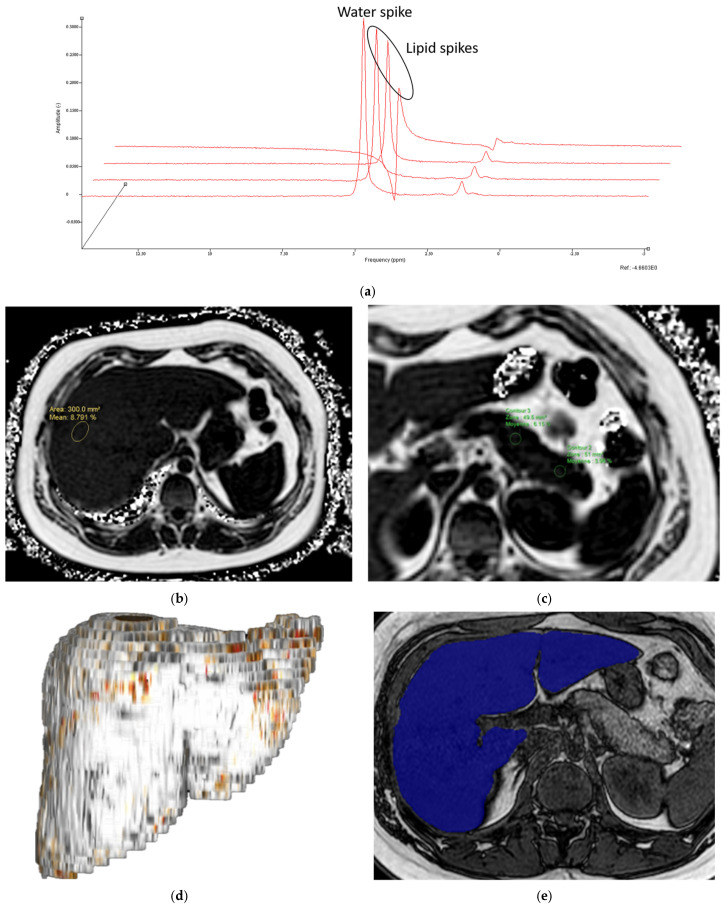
A 15-year-old female with (a) 8.69% liver steatosis measured by MRS and (b) 8.79% liver steatosis measured by MRI-PDFF using the mDixonQuant sequence. This same participant shows (c) pancreas steatosis of 3.98% in the tail and 6.15% in the body. (d) Volume reconstruction of the liver. (e) A single axial slice of the post-processed image used to calculate a liver volume of 2326.2 cm^3^ for the same participant. The same post-processing method generated pancreatic volume.
